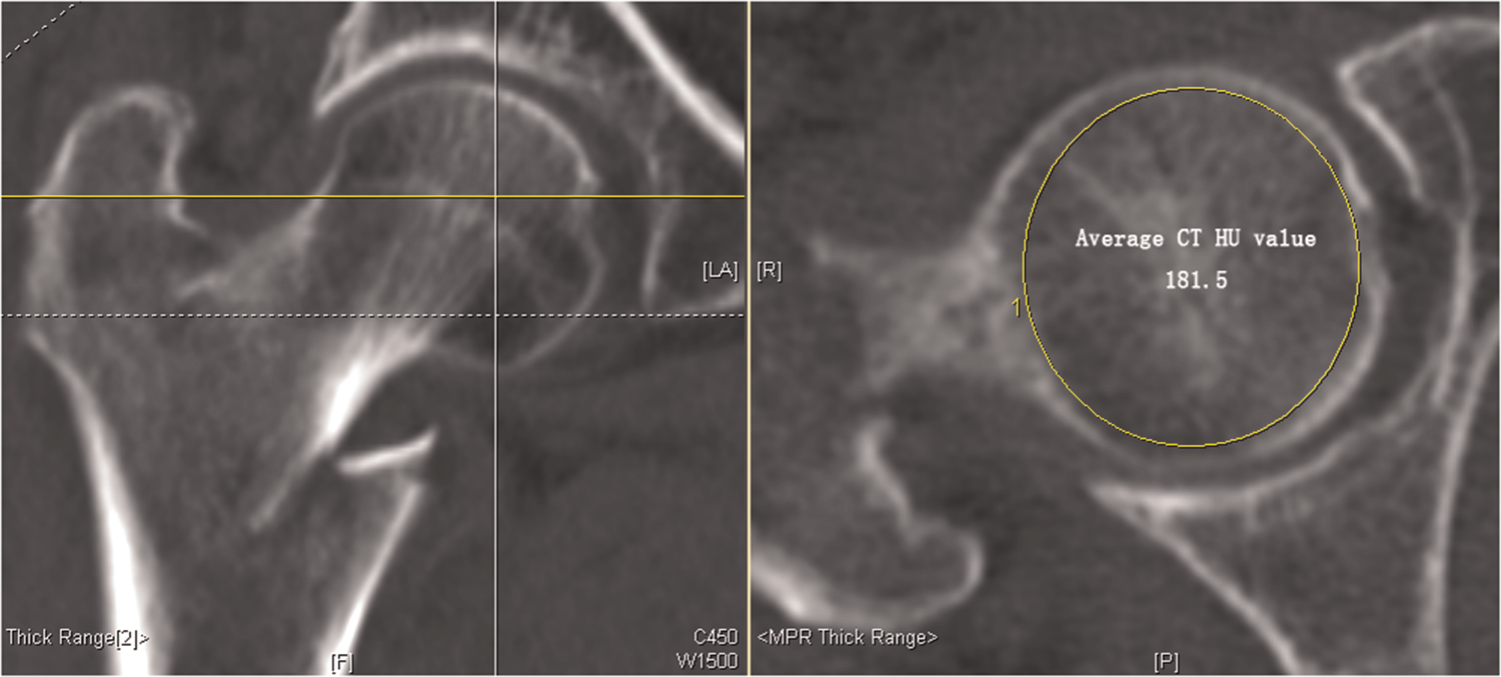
The methods of measuring computed tomography (CT) Hounsfield units (HU) values of the femoral head. A single axial femoral head level was selected. The level included the last slice of femoral neck–first slice of femoral head combination. A spherical region of interest (ROI) was drawn with the largest possible diameter excluding the cortical bone in femoral head and average HU value (181.5 HU) was noted. CT, computed tomography; ROI, region of interest.
